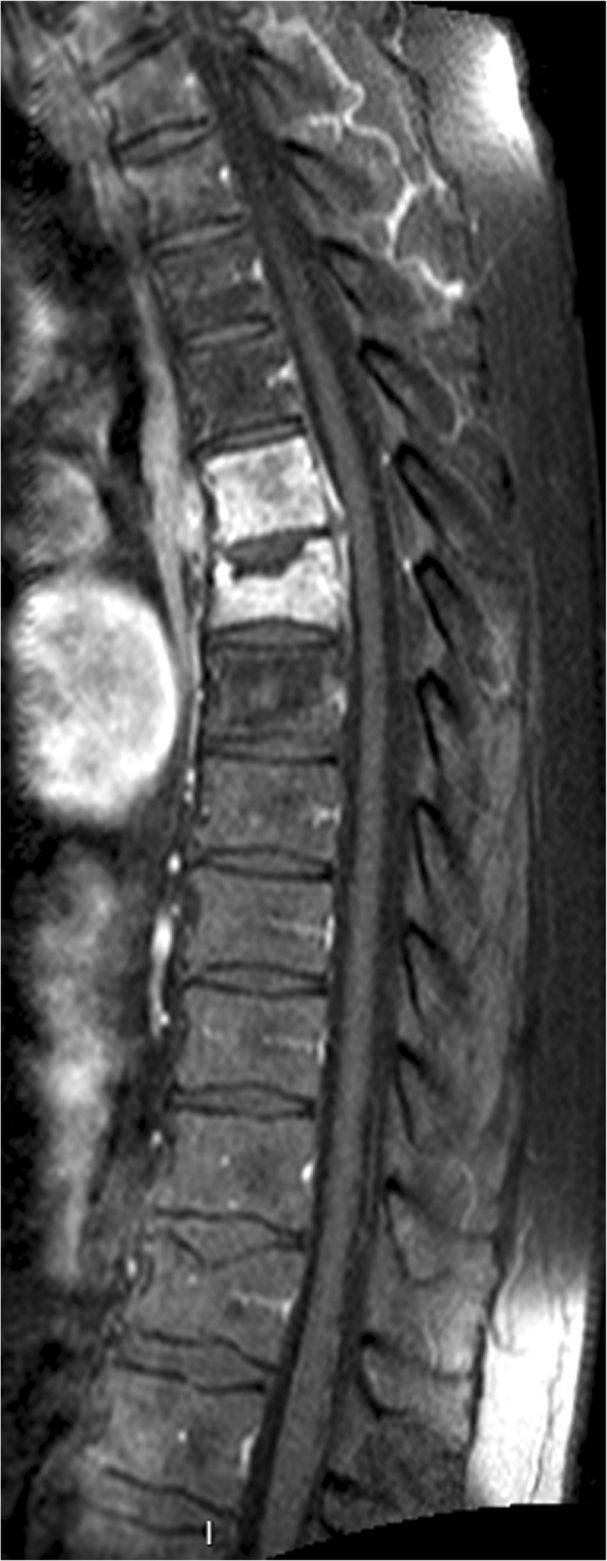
Spine MRI shows fracture settlement of the sixth thoracic vertebra with infiltration from the fifth to the sixth thoracic vertebra
